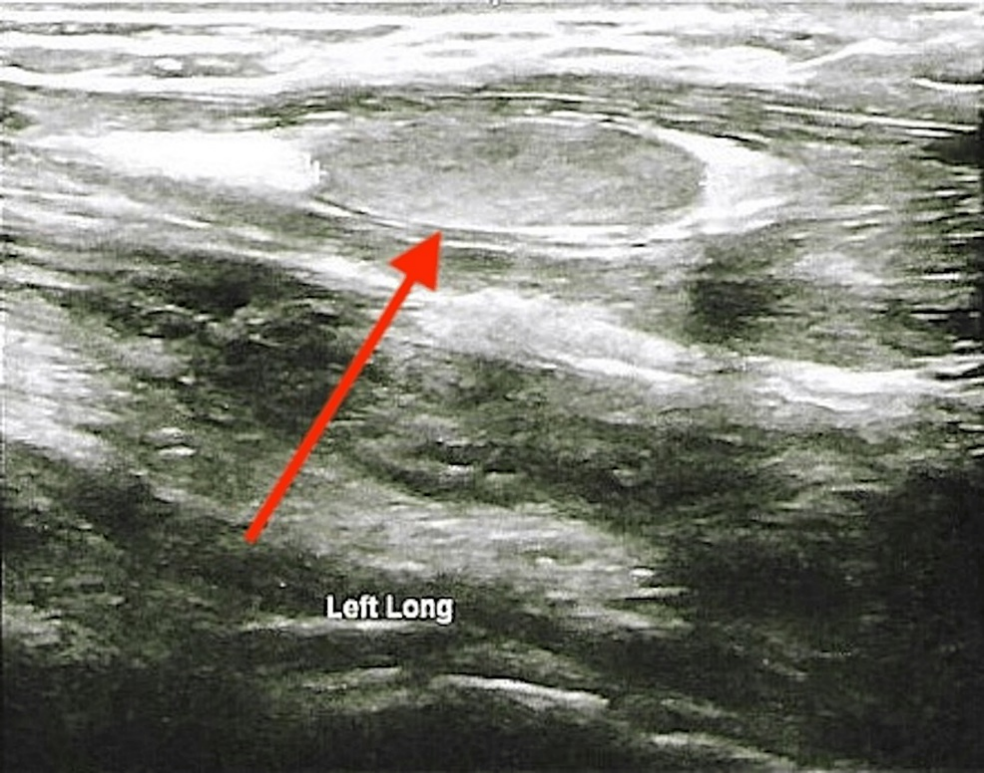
Left forearm ultrasound longitudinal view revealing an auto-transplanted parathyroid adenoma (red arrow)
